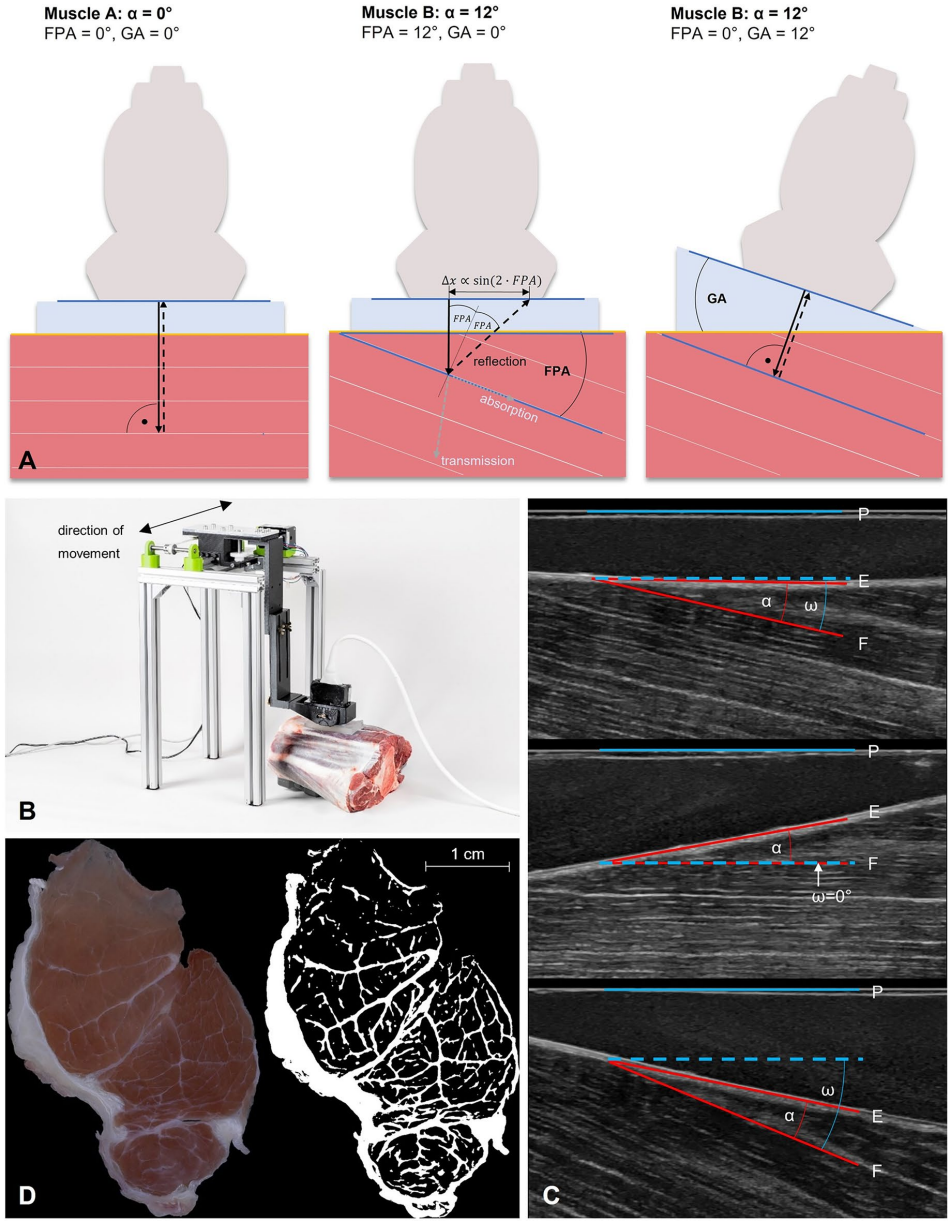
Visualization of ultrasound image acquisition and analysis. (A) The fascicle probe angle (FPA) is subject to variation in both the pennation angle (α, middle) and the gel pad angle (GA, right). Only GA can be controlled by the operator, while α stays constant in a resting muscle. The ultrasound gel pad enables angulation affording minimal ultrasound attenuation prior to coupling into the tissue. At a muscle/IMCT interface, reflection, transmission, absorption, and scattering (not indicated) occur and change with the FPA. A change in FPA causes a change in the angle of reflection x =|FPA|, and hence causes the reflected ultrasound ray to be displaced by ∆x ∝ sin(2 $$\cdot$$|FPA|) in the probe. Thus, a change in FPA will cause all reflected rays within a detector area of fascicle-transverse length ∆x to be reflected away from the probe, and thus the MGV decreases by an amount ∝ ∆x. The image in the middle exemplifies the trigonometric relationship between the probe angle and the amount of ultrasound waves being reflected back to the probe. The echo intensity detected is determined by the function y = β_0_ – (β_1_/2) ∙ sin(2|FPA|) where β_0_ is the mean gray value at 0° FPA (MGV_00) and β_1_ is the tilt echo gain (TEG). (B) Set-up for ultrasound image acquisition: The linear probe is held in place by a probe holder that is attached to a robotic axis translating the probe in the shown direction. (C) Representative ultrasound images of an infraspinatus muscle scanned with a 0° (left), + 12° (middle) and − 2° (right) gel pad. α is the pennation angle, between fascicle (F) and epimysium (E). ω is the fascicle probe angle (FPA), between fascicle and probe (P). The mean gray value is visibly higher at a smaller FPA (middle < top < bottom). (D) Segmentation of macroscopic photo of muscle cross section (left) with *ilastik* (right).
